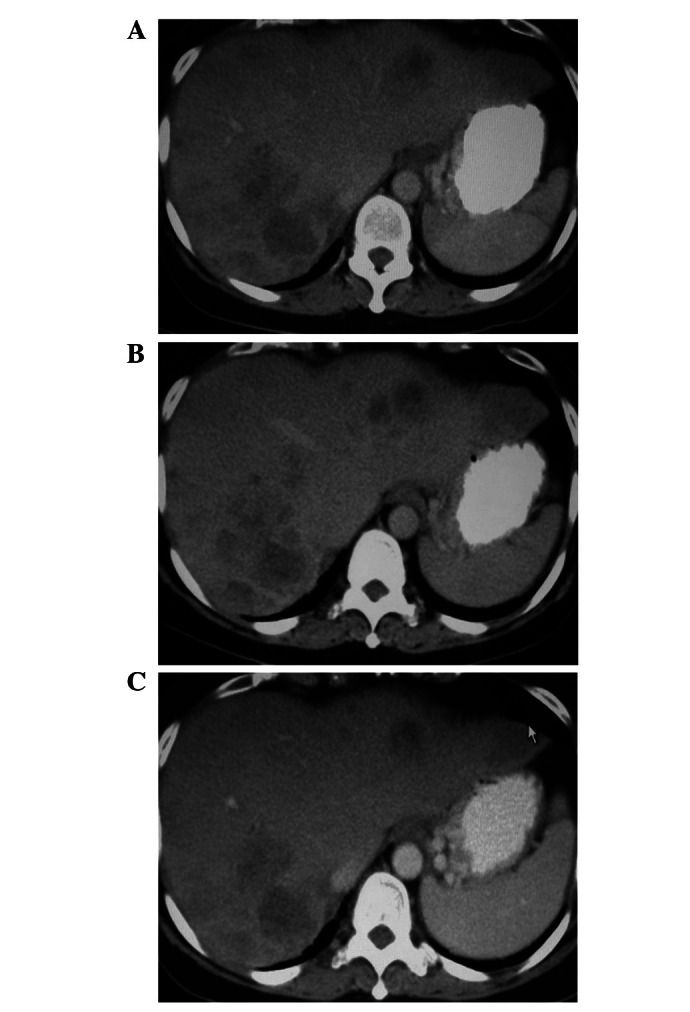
CT scans showing regression of the lesion following AIET infusions. (A) Lesion before infusion, (B) after the third infusion and (C) after the sixth infusion. AIET, autologous immune enhancement therapy.
